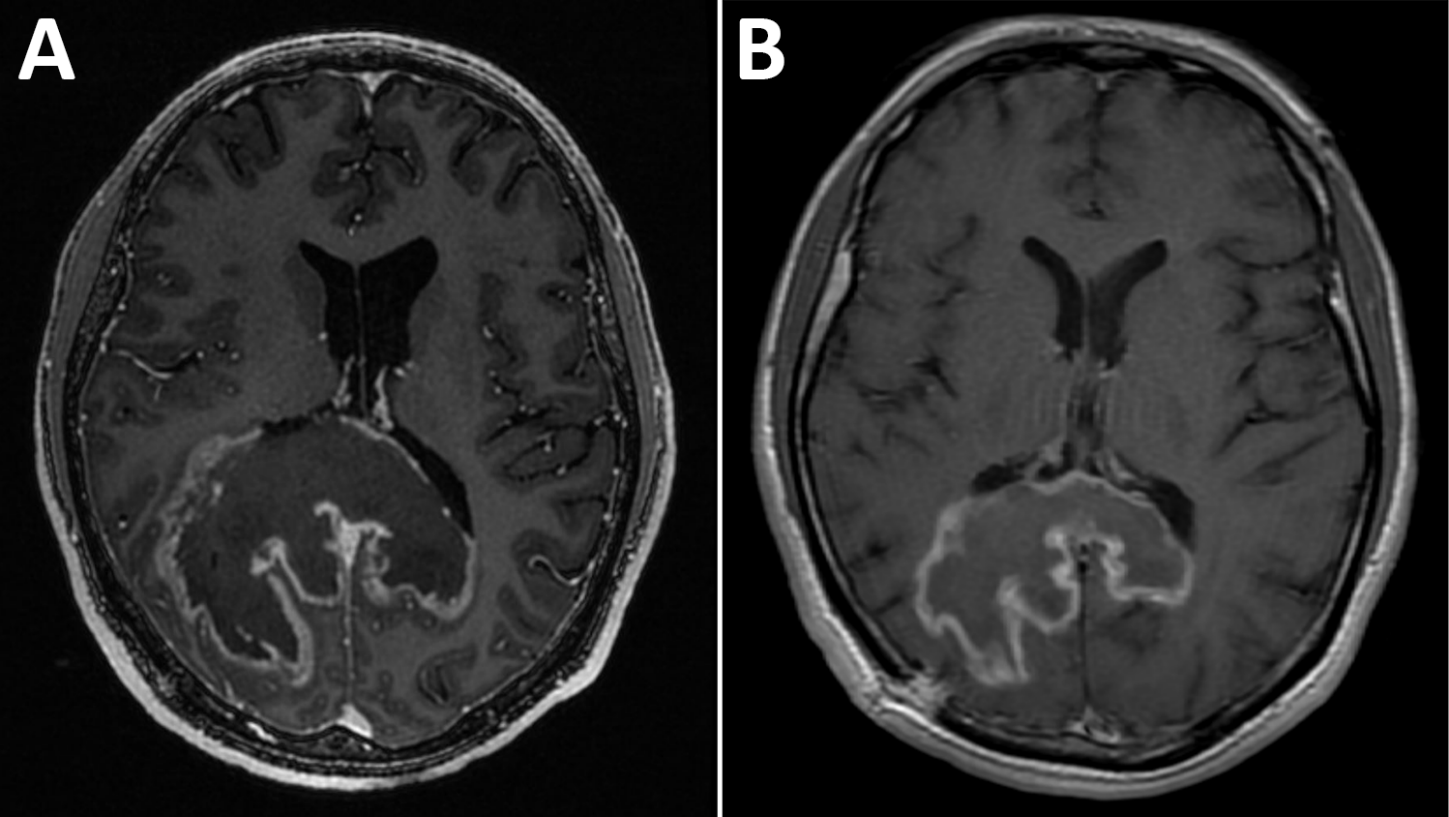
Images obtained during diagnosis of chagasic encephalitis in 31-year-old man in the United States. A) Contrast-enhanced T1-weighted magnetic resonance imaging of the brain showing a cerebral tumor-like chagoma in the axial plane. B) Follow-up contrast-enhanced T1-weighted magnetic resonance imaging obtained ≈8 weeks later showing improvement of the chagoma.
